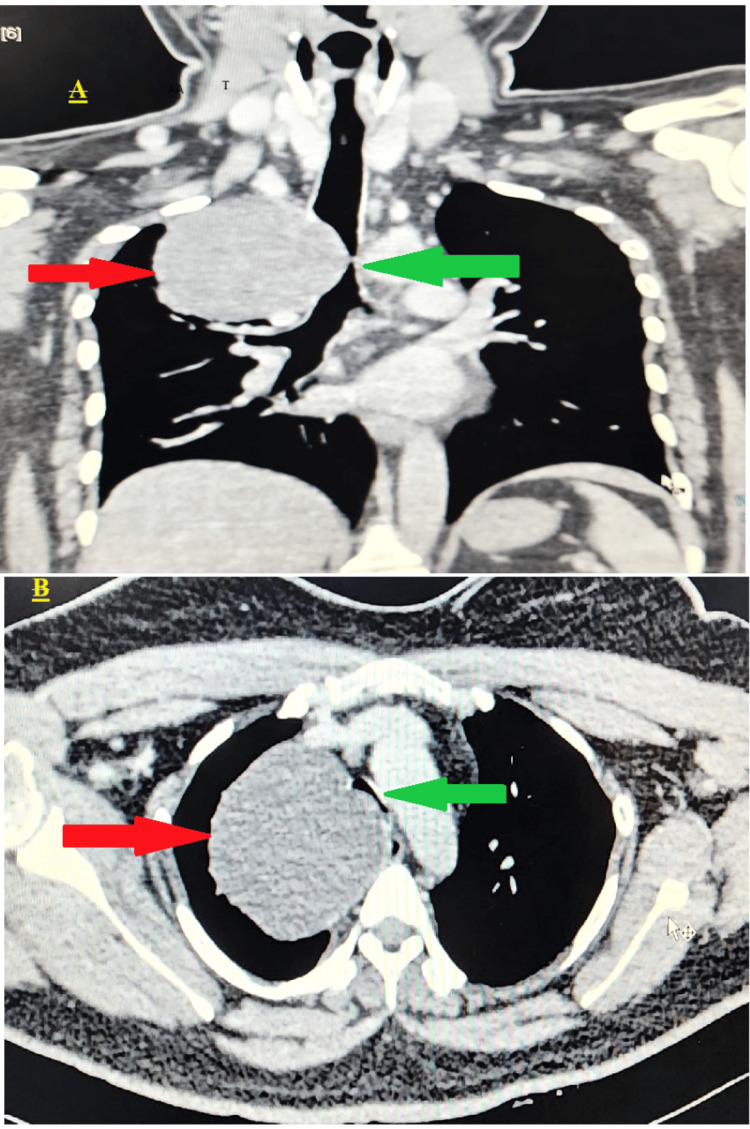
CT thorax. (A) Coronal section (red arrow showing large BC in the paratracheal region of the superior mediastinum and green arrow pointing toward severe tracheal compression of the lower one-third of the trachea). (B) Axial section (red arrow showing BC and green arrow showing severe tracheal narrowing). BC, bronchogenic cyst
